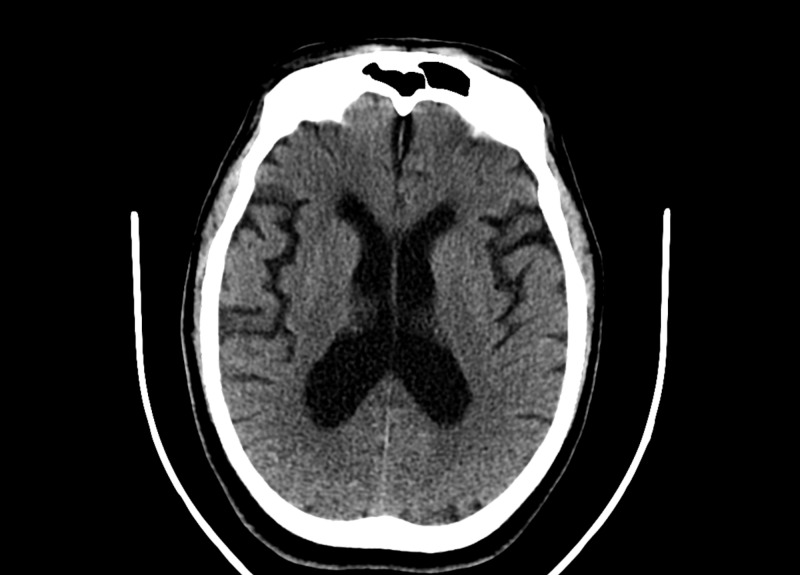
Computed Tomography of the Brain Without Contrast (axial view)
